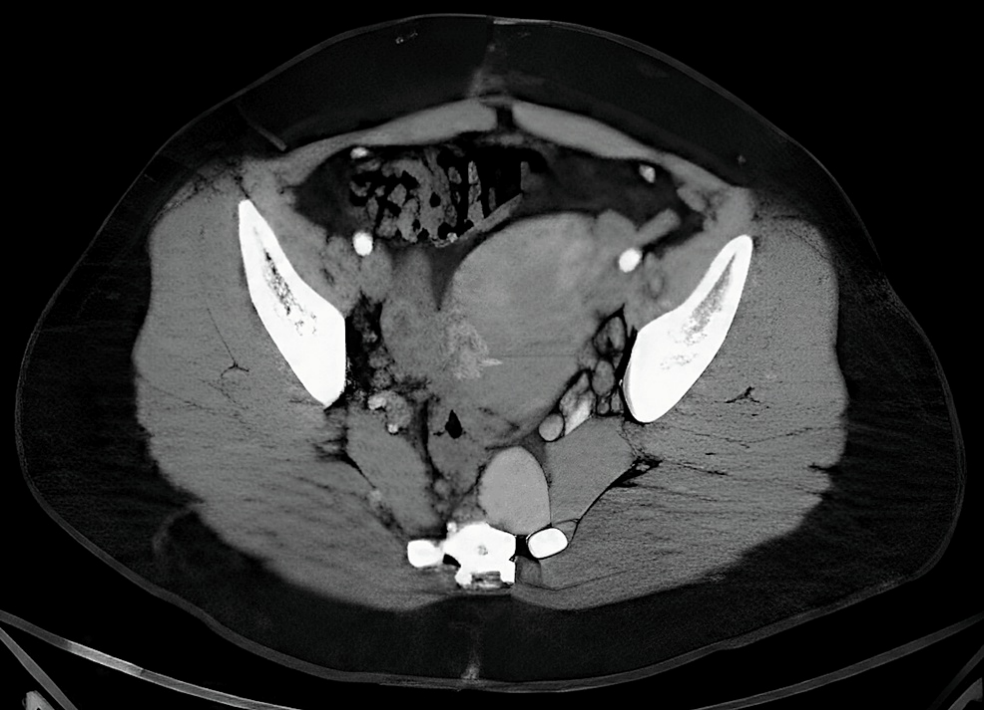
A CT scan of the abdomen and pelvis showed a homogeneous, solid left perirectal lesion.
